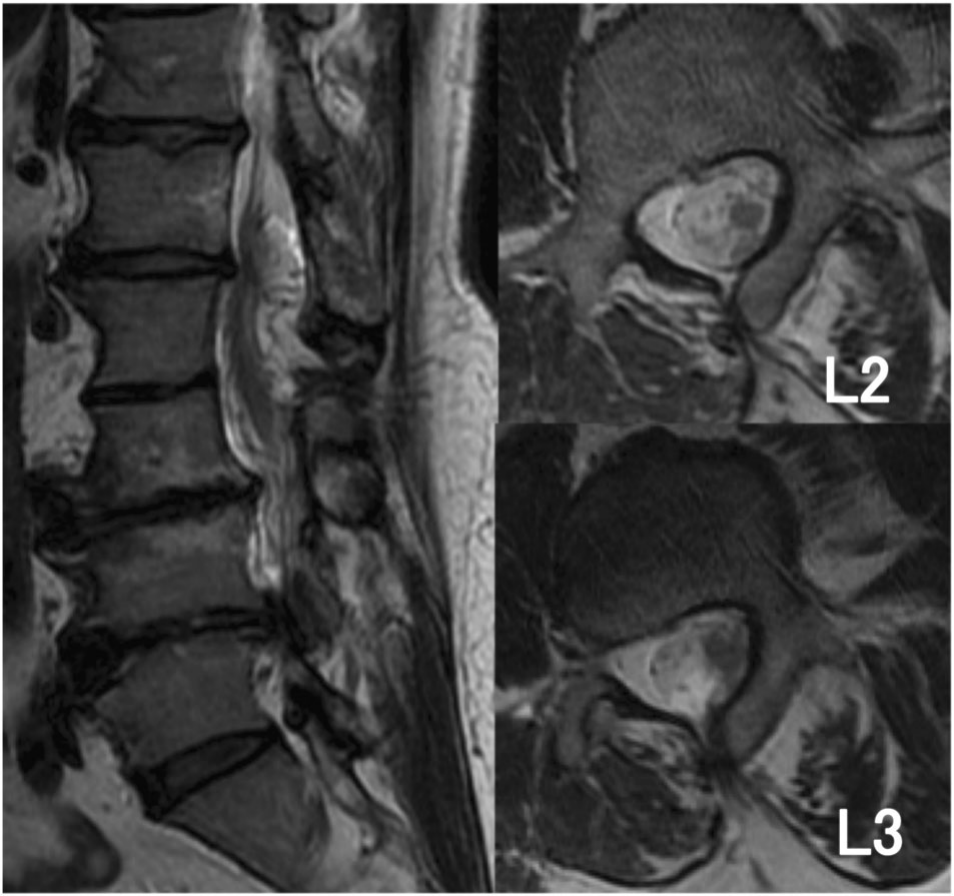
Case 2. T2-weighted MRI scans showing a spinal lipoma at the L2/3 level with a flow void dorsal to the spinal cord
